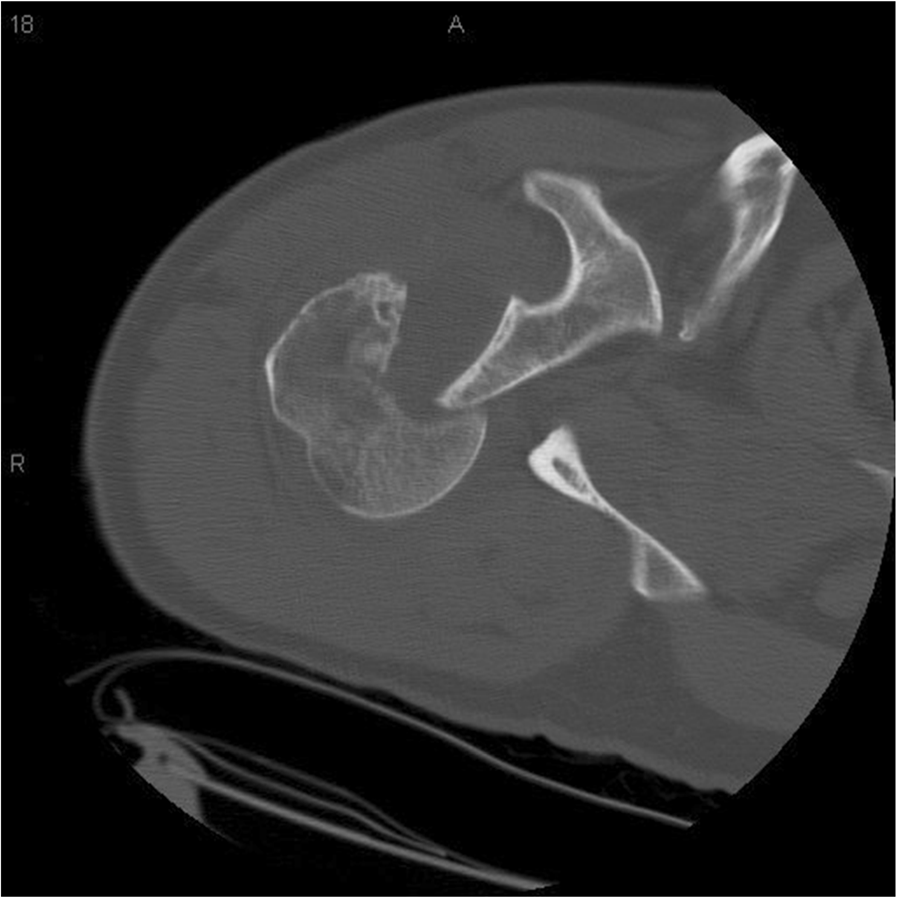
CT scan of the right shoulder axial view showing the posterior dislocation and reverse Hill Sachs lesion.
